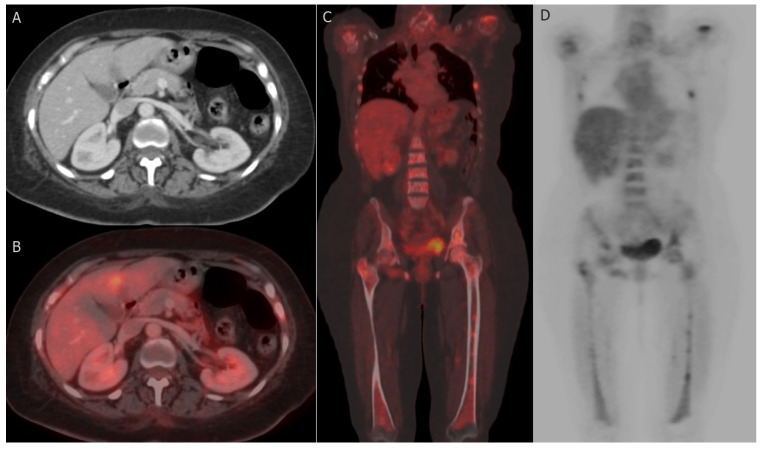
Hepatic and bony metastases: 61-year-old woman with grade II invasive ductal carcinoma who underwent a right modified radical mastectomy. (A,B) axial post-contrast CT image and fused FDG PET/CT image reveal FDG-avid hepatic metastatic deposit at segment IV b with a maximum standardized uptake value (SUVmax) of 8.5. (C,D) coronal-fused FDG PET/CT and maximum intensity projection (MIP) images reveal FDG-avid multiple bony metastatic deposits at ribs, proximal humeri, lumbar vertebrae, iliac bones, and both femori with SUVmax of 9.8.
